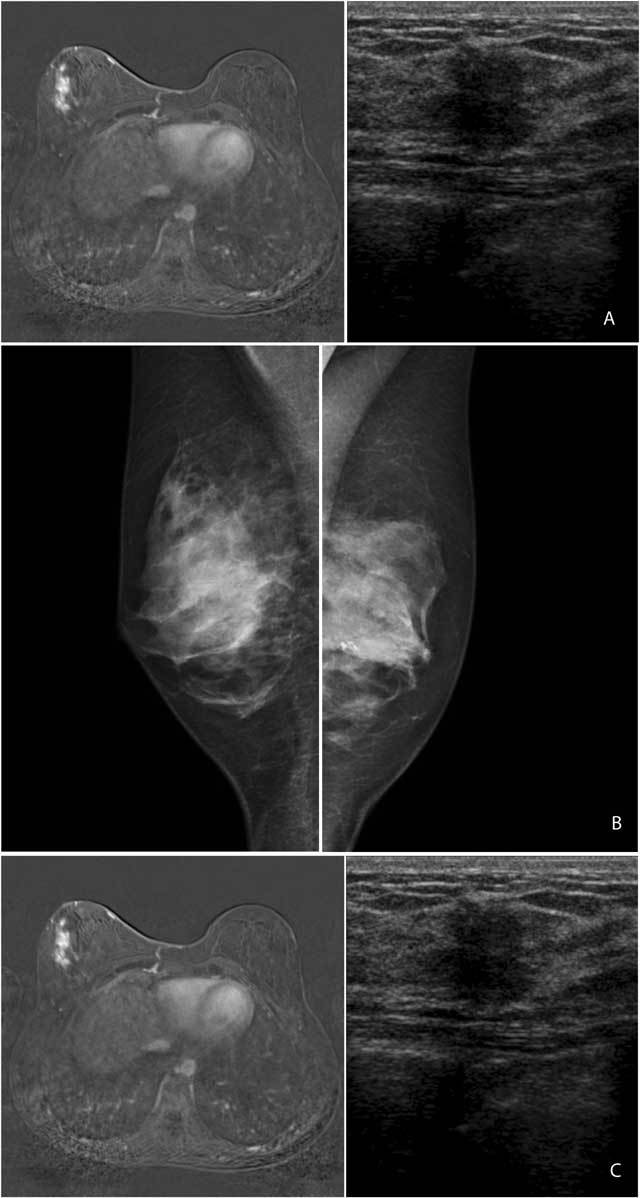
2012: Mammogram of the right breast shows no peculiarities; in the left breast there is a macrocalcification due to liponecrosis due to surgery (A). MRI of the breasts, T1 substraction image after contrast administration shows ill-defined multicentric nodular contrast enhancement in the left breast (B). Ultrasound of this region shows an ill-defined hypoechoic mass with mild posterior acoustic shadowing (C).
